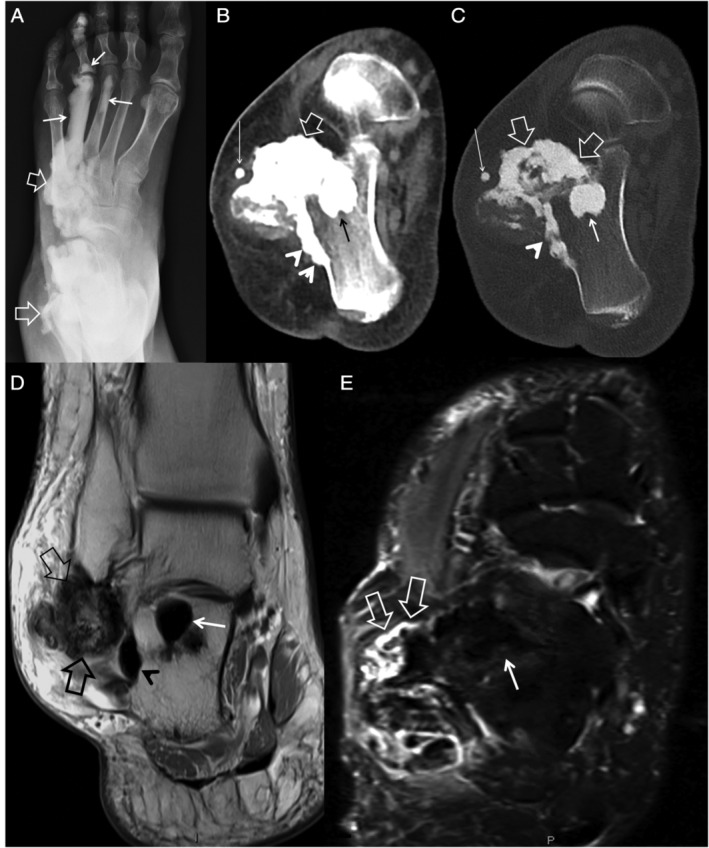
MRI in melorheostosis. A 42‐year‐old female (Melo‐4) patient with a follow‐up of 7 years after initial diagnosis. Plain radiograph (*A*) of the foot showing endosteal hyperostosis (thin arrows) and parosteal lesions (open arrows), not well‐defined because of overlapping structures. Axial CT images in the soft tissue (*B*) and bone window (*C*) show endosteal hyperostosis within the os calcis trabecular bone (arrows), the parosteal lesion on the lateral calcaneal cortex (arrowheads) and new bone formation of “dripping candle‐wax” pattern extending into the tarsal sinus (open arrows). Small soft tissue ossification (long thin arrow) is also shown. Coronal proton‐density–weighted (*D*) and axial fat‐suppressed T2‐ weighted (*E*) MR images showing the low signal endosteal hyperostosis (arrows), the parosteal hyperostosis (arrowhead in *C*), and a dripping candle‐wax lesion caudally to the lateral malleolus (open arrows) in greater detail as compared with CT. On the fat‐suppressed image, the dripping candle‐wax lesion is surrounded by a high signal‐intensity–reactive tissue (open arrows in *C*).
